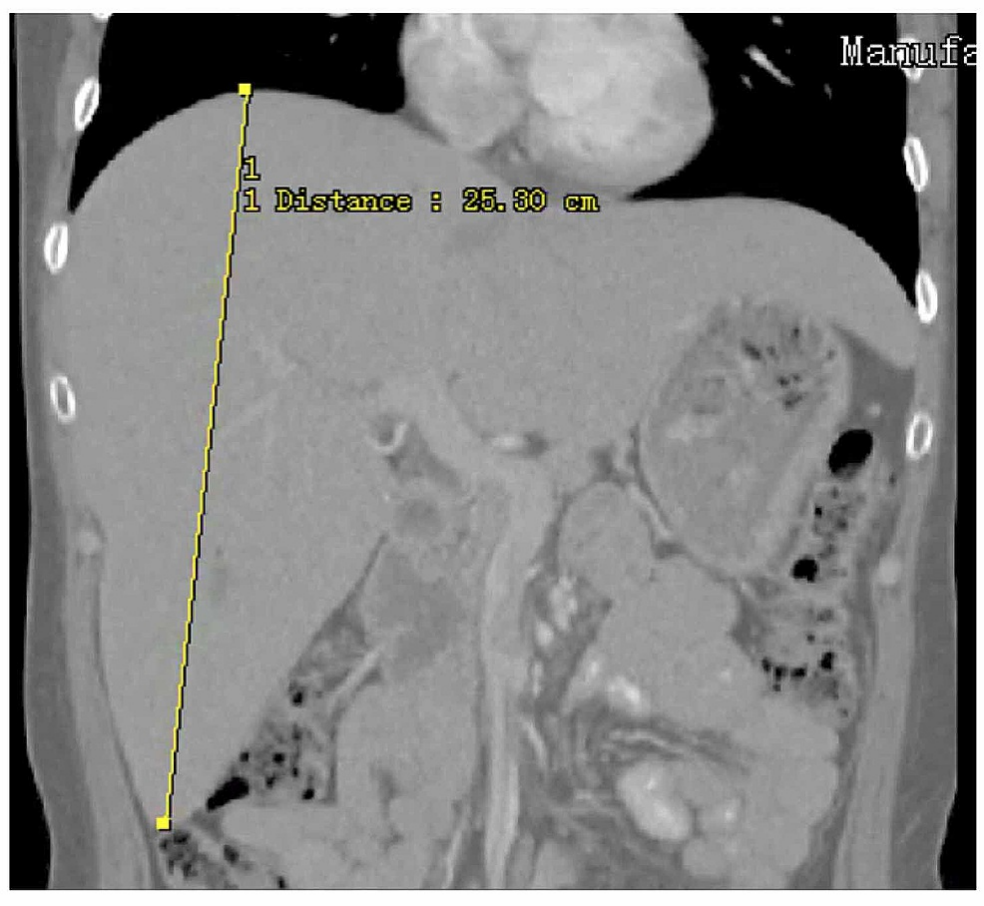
MRI of the abdomen demonstrating markedly enlarged liver during one of the patient's hospitalizations
